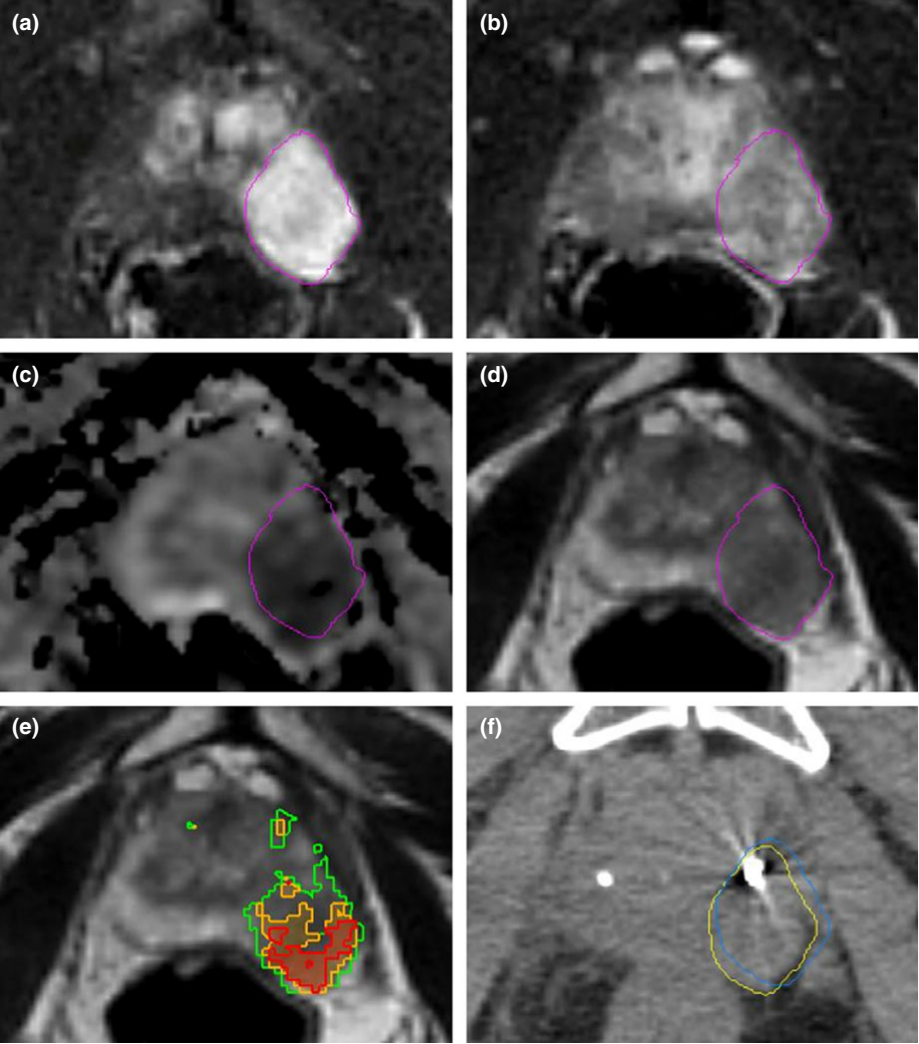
DIR and GTV delineation on mpMRI. (a) Early phase of DCE‐MRI; (b) Late phase of DCE‐MRI; (c) Apparent diffusion coefficient map; (d) T2‐image showing the physician defined dominant tumor, GTV; (e) Probability map of dominant tumor location based upon analysis of mpMRI; (f) (planning CT) Physician defined dominant lesion has been transferred to the CT using a rigid registration (blue) and a deformable registration (yellow). Note how the contour transferred using the DIR follows the anatomy of the CT scan more closely than the rigidly transferred contour.
